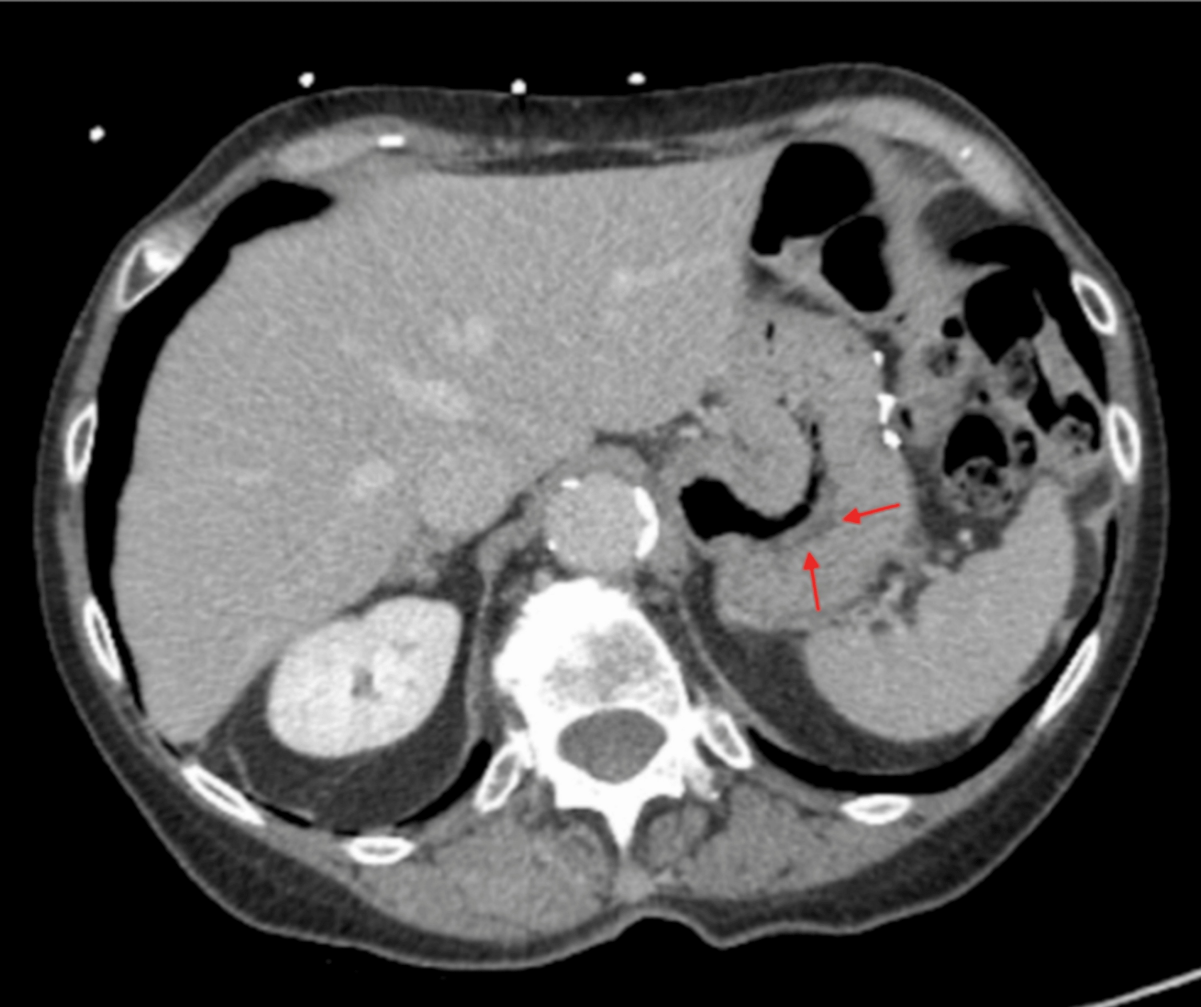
CT scan demonstrating gastric wall thickening and no active bleeding (shown by red arrows) CT, computed tomography
